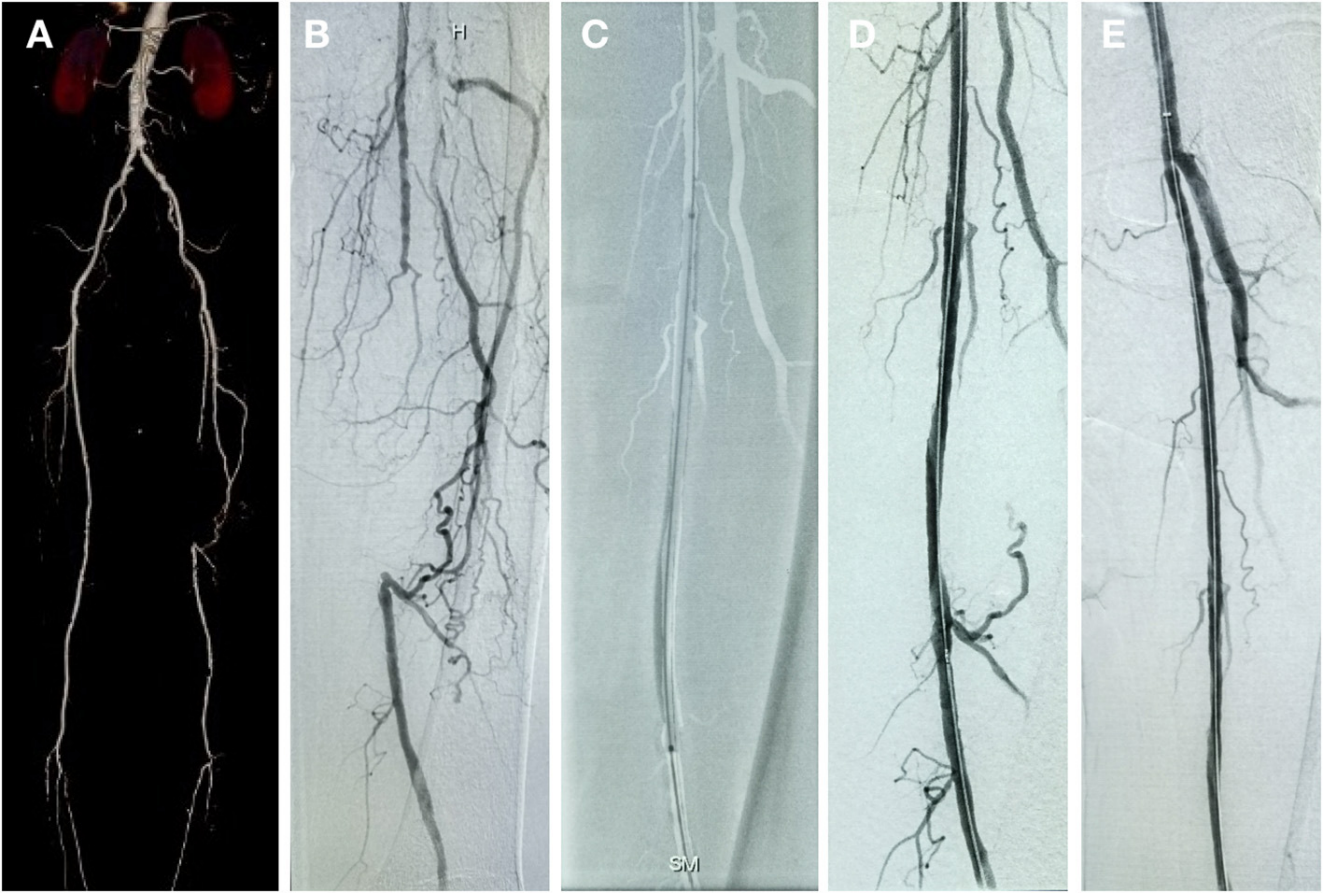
Patient with chronic total occlusive lesion in the left SFA treated with DCB and follow-up result. (A) Preprocedure computed tomography angiography (CTA). (B) Initial angiogram during procedure. (C) Inflated balloon. (D) Postprocedure angiogram. (E) 6-month follow-up angiogram showing no restenosis of the target lesion.
